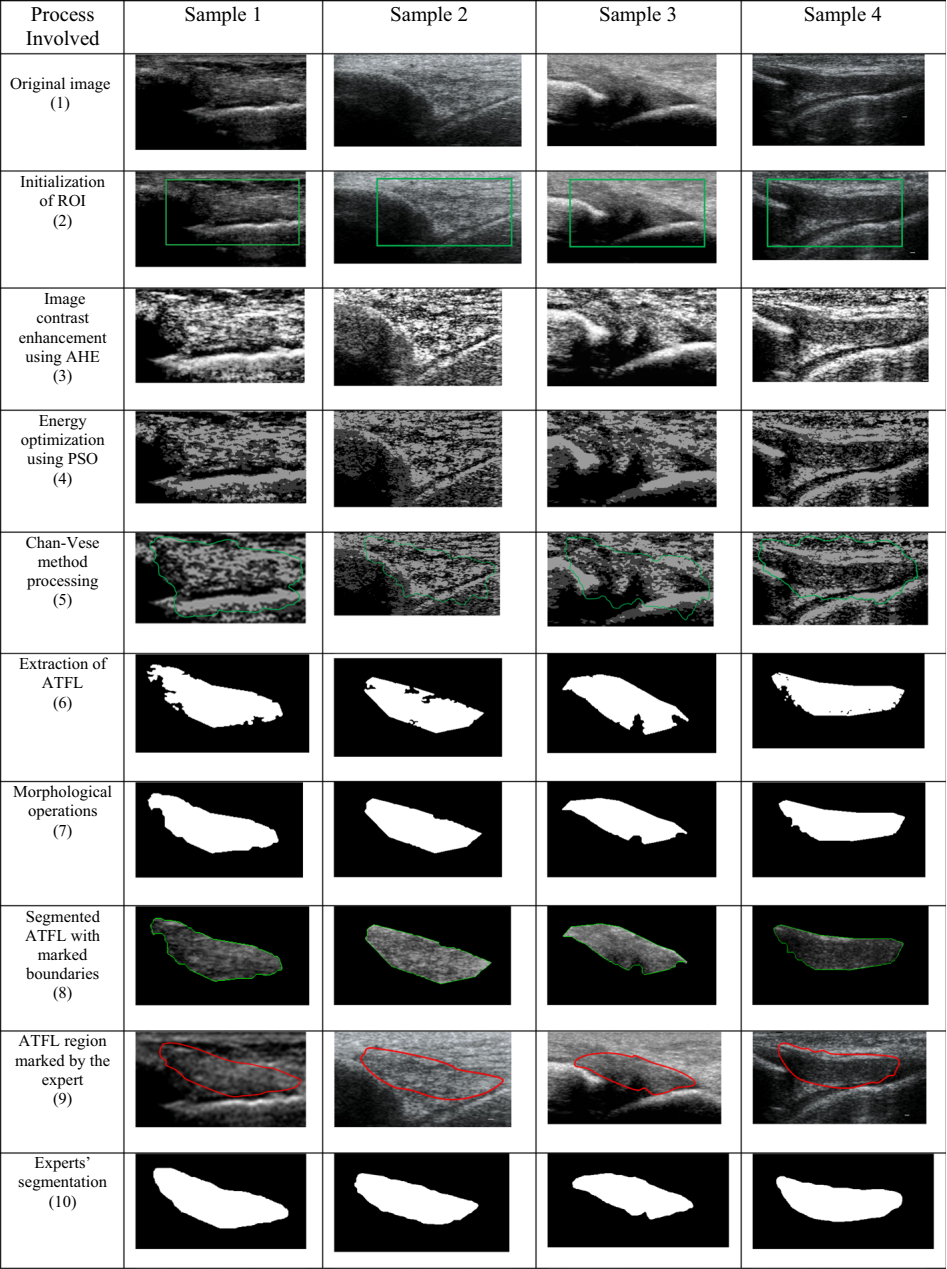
ATFL segmentation from 2D ultrasound images: steps involved (*first column*) and corresponding outcomes at each stages of developed framework for four different samples images (*column 2–5*)
